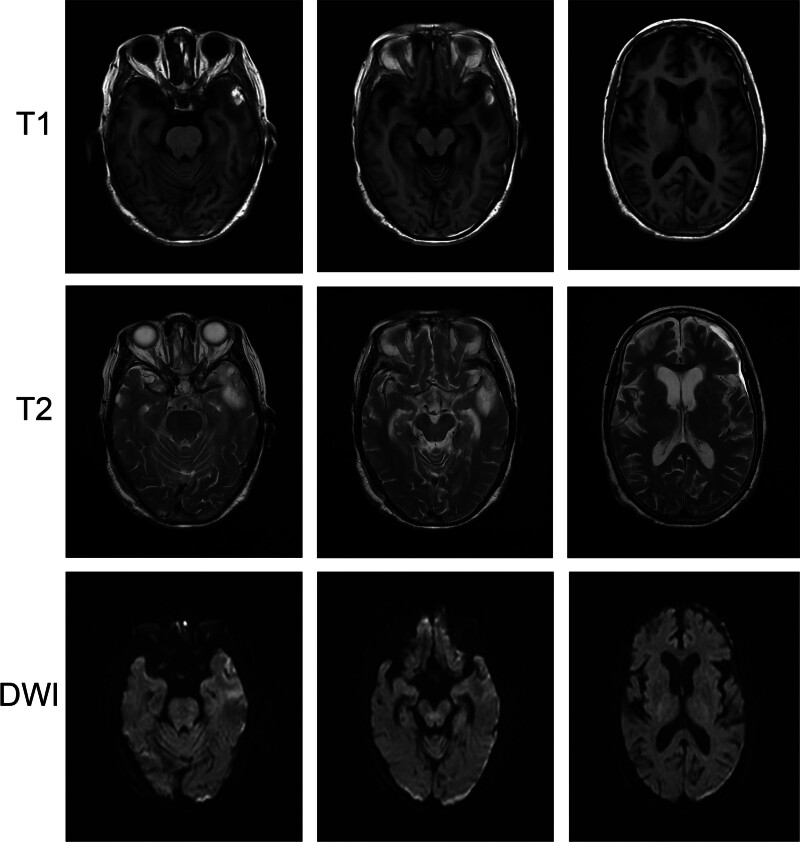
Head MRI image after considering Guillain–Barré syndrome. Left temporal band of long T1 and T2 signal shadows with high signal on DWI. DWI = diffusion-weighted imaging, MRI = magnetic resonance imaging.
